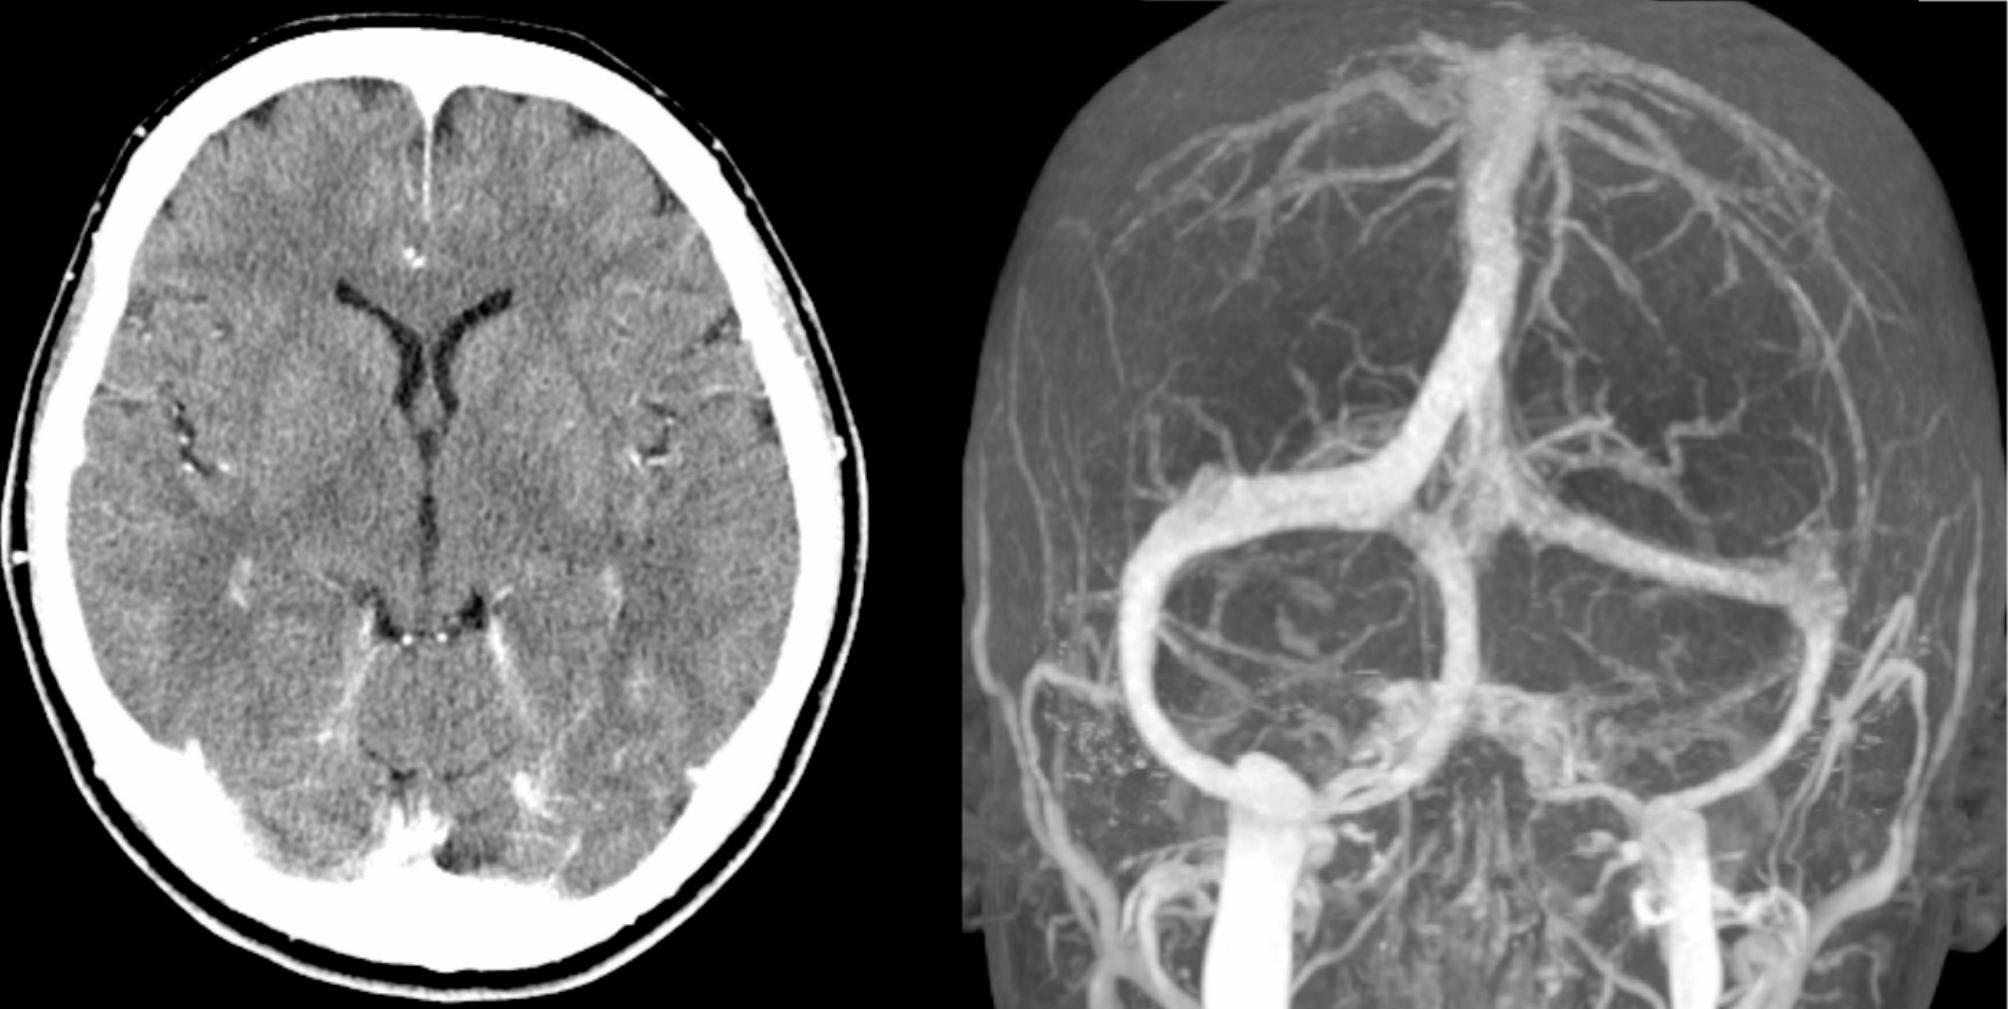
Computed tomography and computed tomography venography in the case. There were no abnormalities in the brain parenchyma, and no venous occlusion
